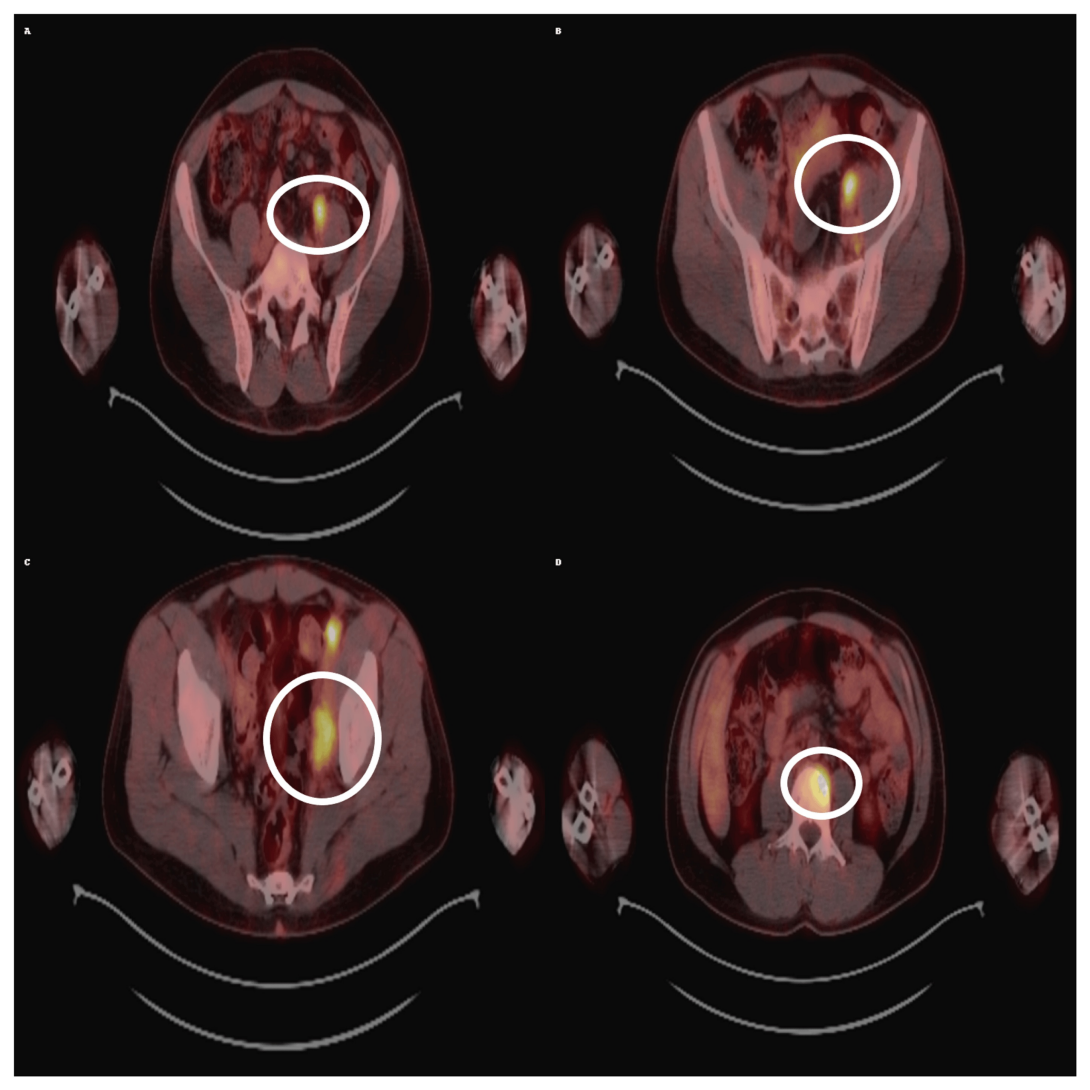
Baseline PET/CT scan showing hypermetabolic lesions in the pelvis and spleen. Axial fused PET/CT images (A-D) demonstrate increased uptake consistent with Kaposi sarcoma involvement. The regions of interest are indicated by the white ellipses, with the maximum standardized uptake value (SUVmax) ranging between 3.9 and 6.4 at this stage.
